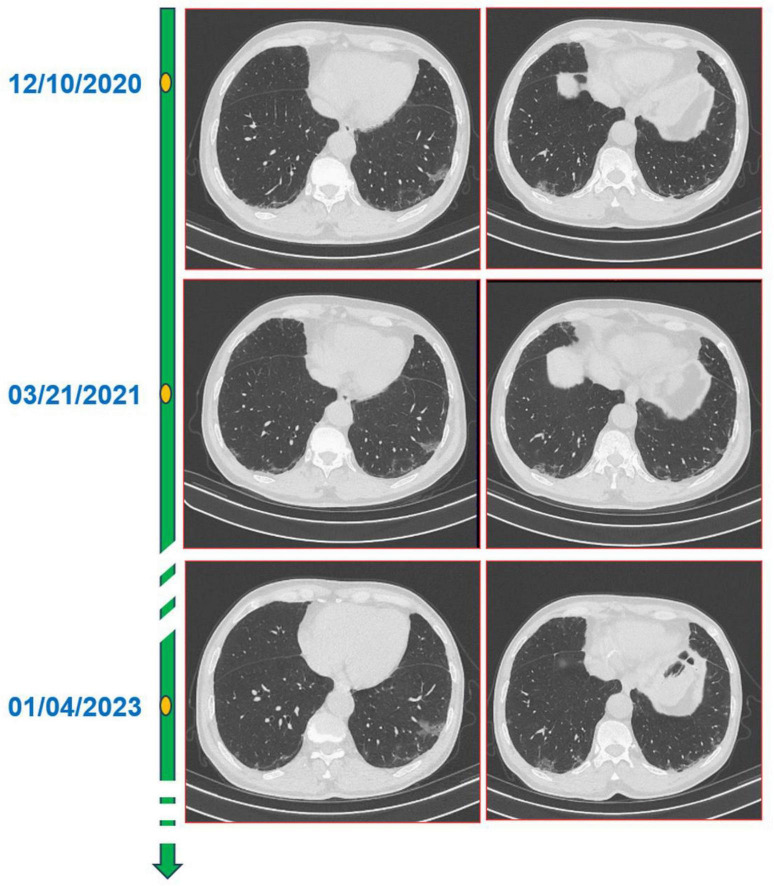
A total of 16-slice computerized tomography images of the chest at initial diagnosis period and in the subsequent 2 years of annual chest computed tomography (CT) re-examination of the patient.
